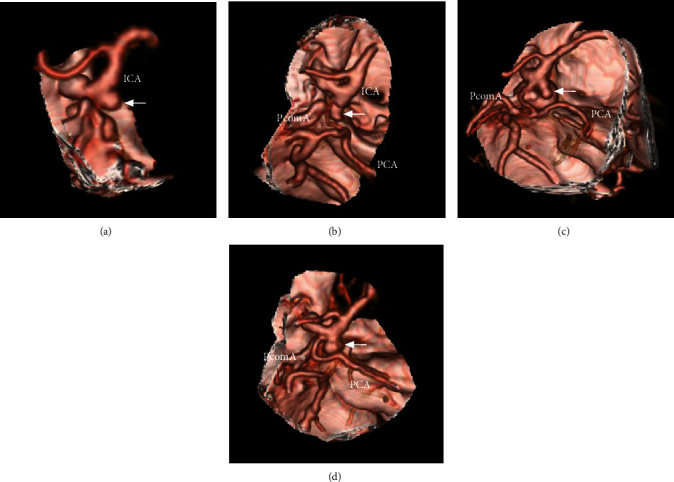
PcomA development as shown by CTA is as follows: (a) Grade I is defined by the absence of the PcomA; the arrow indicates the aneurysm. (b) Grade II is defined by the presence of a thin PcomA (asterisk); the arrow indicates the aneurysm. (c–d) Grade III is defined by the presence of a thick fetal-type PcomA (asterisk); the arrow indicates the aneurysm. Abbreviations: CTA: computed tomography angiography; ICA: internal carotid artery; PCA: posterior cerebral artery; PcomA: posterior communicating artery.
